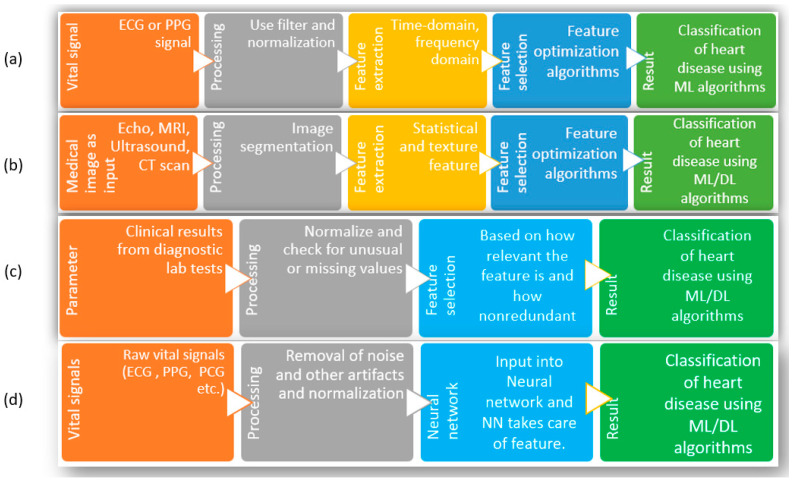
Different methods of heart disease classification using ML and DL. (a) Input as vital signs and extraction of features, (b) input as images (Echo, MRI, ultrasound, CT scan), (c) input as clinical parameters, (d) input as raw vital signs.
